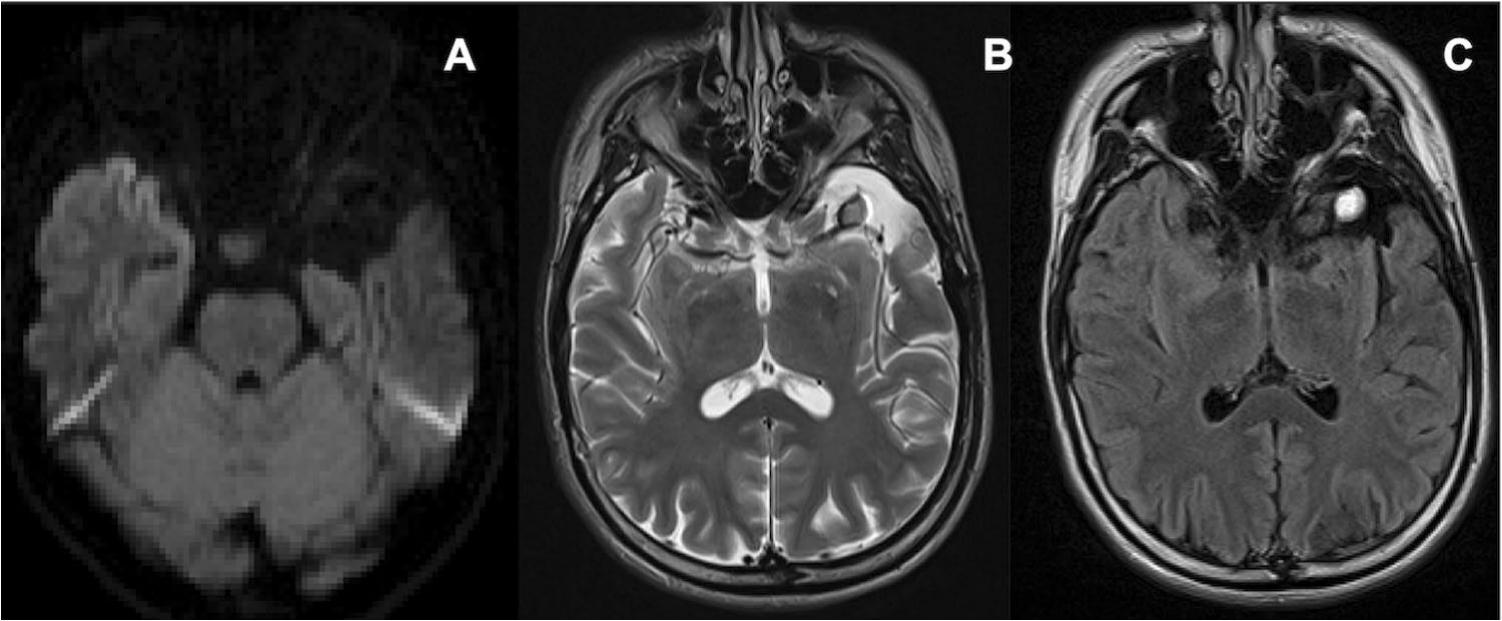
After the anterior temporal lobectomy, the Sylvian lipoma is shown isolated from the temporal lobe on axial diffusion-weighted (A), T2-weighted (B), and fluid-attenuated inversion recovery imaging (C)
